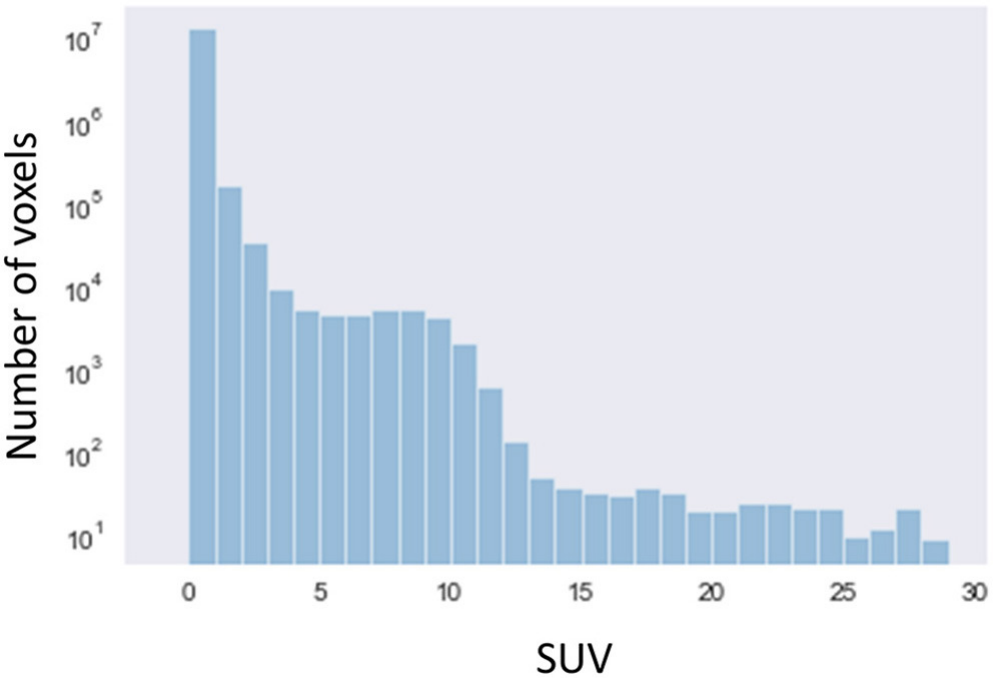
A histogram of SUV over the whole-body image of a patient (semi-log plot).
